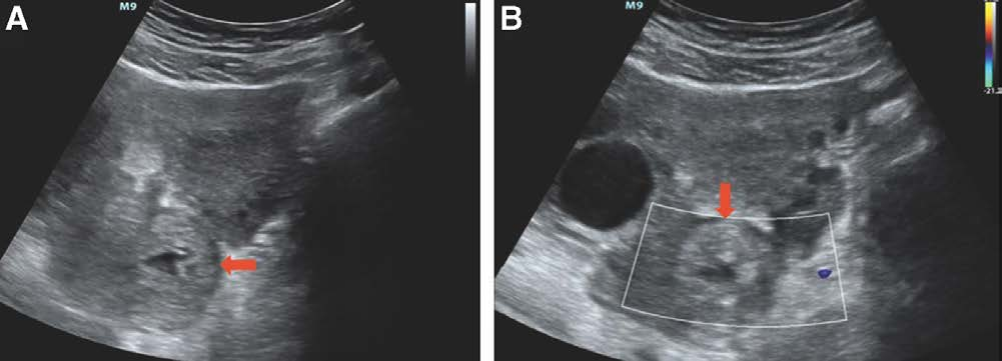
Abdominal ultrasound reveals a gestational sac with thick echogenic margins in the posterior to the uterus. (A) No signals of blood flow is shown within the gestational sac by color Doppler flow. (B).
